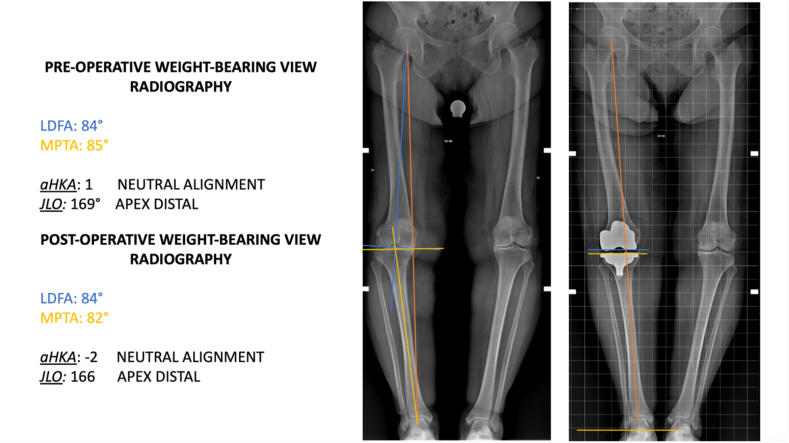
The joint line orientation is parallel to the floor on a weight-bearing radiograph in all patients treated with KA-TKA.
